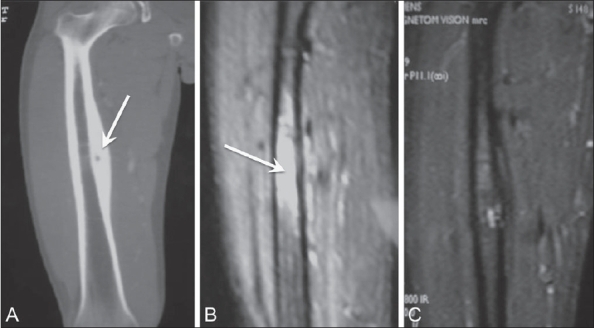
Osteoid osteoma. Pre-ablation CT scan (A) shows the nidus (arrow) of the osteoid osteoma; there is marrow edema (arrow) on a STIR MRI (B). The post-ablation MRI (C) shows complete absence of marrow edema
